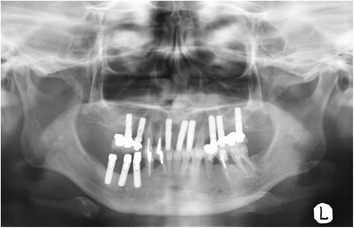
Panoramic radiograph shows slight vertical bone resorption around the implants in the right side of the mandible
